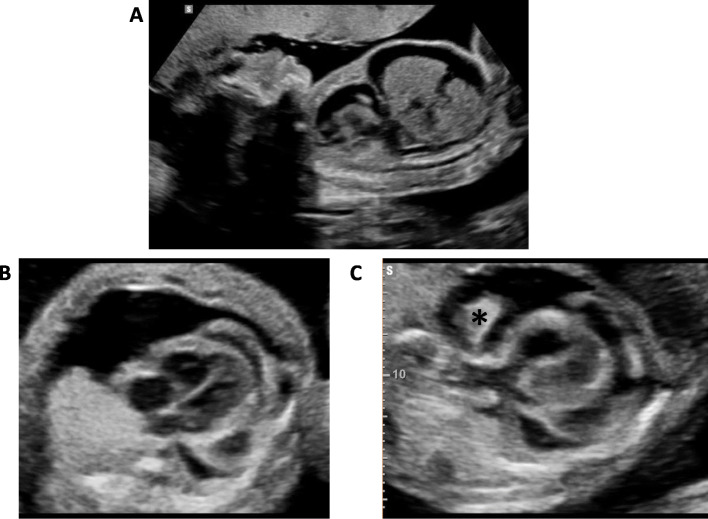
Ultrasound assessment at 25 weeks’ gestation, with evidence of fetal hydrops with ascites and a pleural effusion seen on sagittal view (a), a 4-chamber axial view of the heart shows persisting complex epicardial mass predominately associated with the left ventricle (b) and features suggestive of pseudoaneurysmal rupture with heterogenous material consistent with blood clot (*) seen in the pericardial effusion (c)
